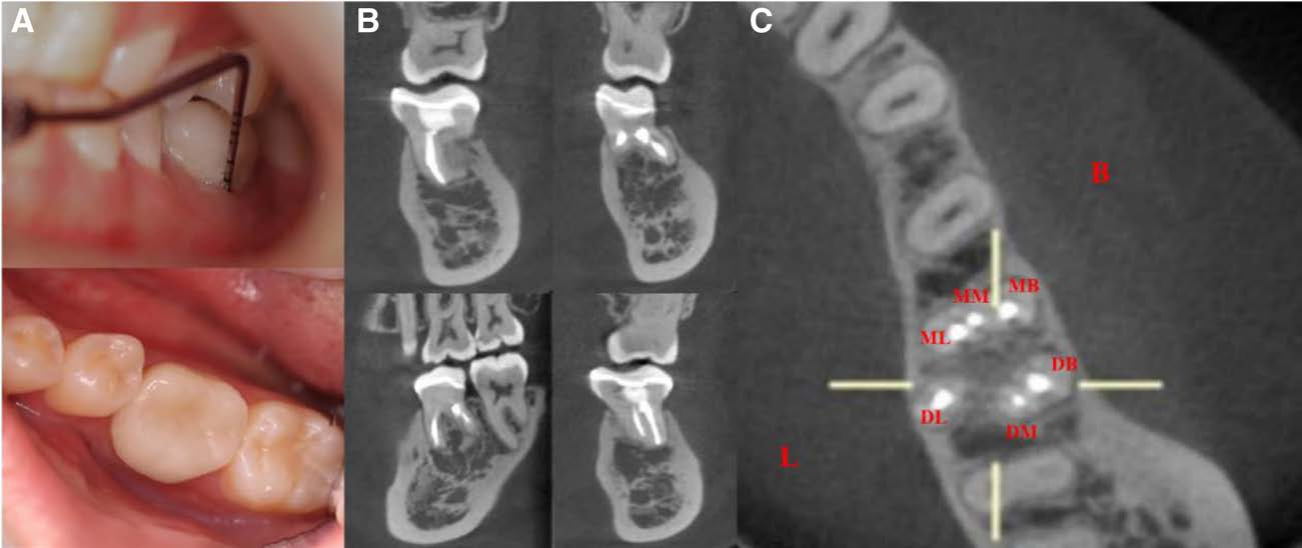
Intraoral photographs and CBCT images of tooth 36 at the one-year follow-up visit. (A) Intraoral photographs. (B onlay C) CBCT images. CBCT = cone-beam computed tomography.
